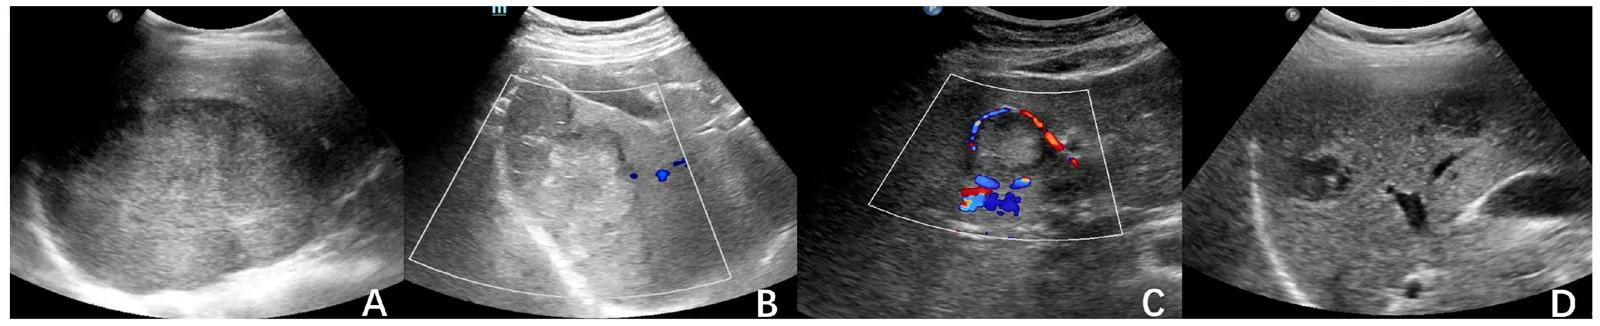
Representative conventional ultrasound images of hepatic metastases following adrenocortical carcinoma resection. (A) Solitary hepatic lesion with well-defined margins and regular morphology. (B) Hepatic lesion with well-defined margins, irregular contour, and absence of detectable vascularity on CDFI. (C) Hepatic lesion demonstrating peripheral rim-like flow signals on color Doppler imaging. (D) Multiple hepatic lesions.
